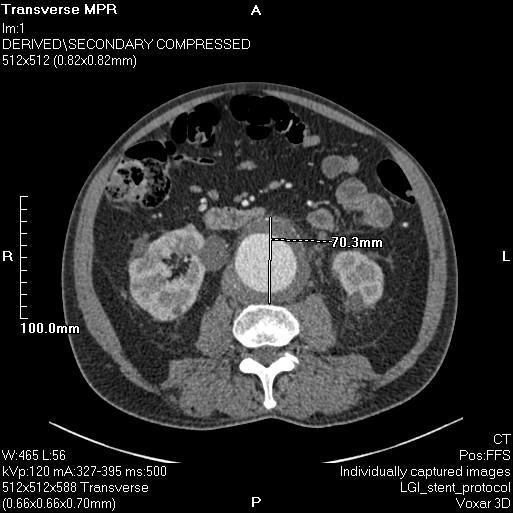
CT aortogram demonstrating a rapid increase in the size of the AAA measuring 7.0 cm in the anteroposterior diameter. There is a new beak in the left lateral aortic thrombus and signs of impending rupture.
